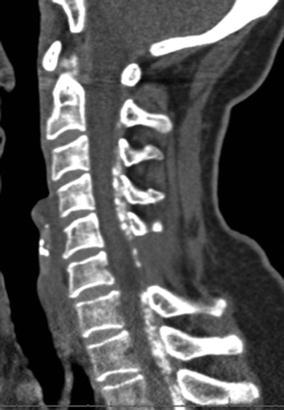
Sagittal CT-scan showing oval nodular densities partially connected with the laminae
